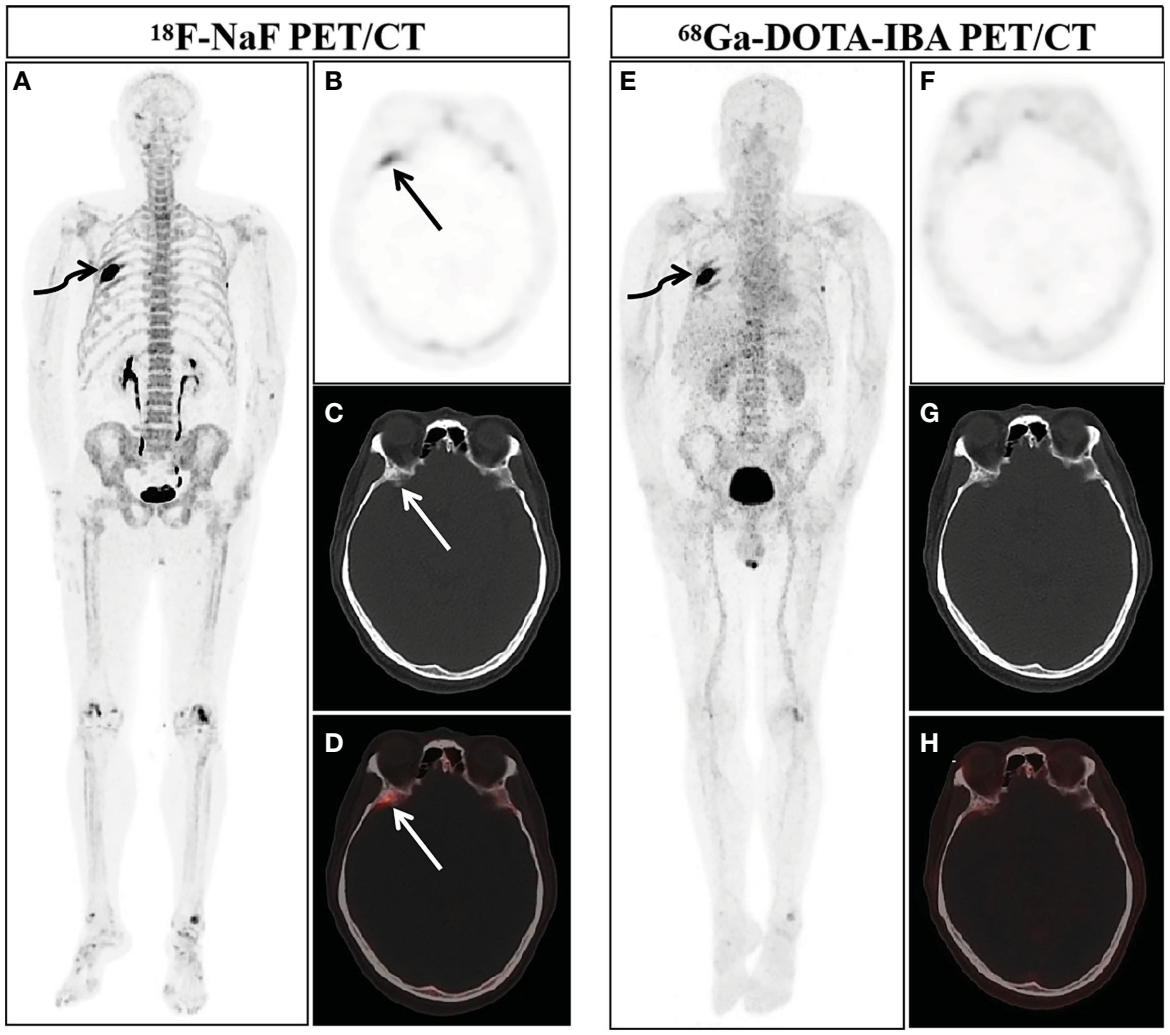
A 58-year-old man newly diagnosed with lung cancer was included in our study and underwent ^18^F-NaF and ^68^Ga-DOTA-IBA PET/CT. The MIP images of ^18^F-NaF (A) and ^68^Ga-DOTA-IBA (E) PET/CT both showed an increase in imaging agent uptake in the right rib (curved arrows). Combined with his history of malignant tumor and typical osteolytic bone destruction with soft tissue shadows, the diagnosis of bone metastasis was considered. The MIP image (A) and axial views (B: PET image; C: CT scan; D: PET/CT fused image) of ^18^F-NaF showed the increased imaging agent uptake of the right sphenoid wing (straight arrow, SUVmax 5.3), but no significant bone abnormalities were observed. ^68^Ga-DOTA-IBA PET/CT MIP (E) and axial views (F: PET image; G: CT scan; H: PET/CT fused image) revealed there was no significant abnormal increase in imaging agent uptake on the right sphenoid wing. After nearly three months of follow-up, according to the patient’s CT examination, there were no obvious abnormalities in the bone, and we believed that this was physiological uptake.
